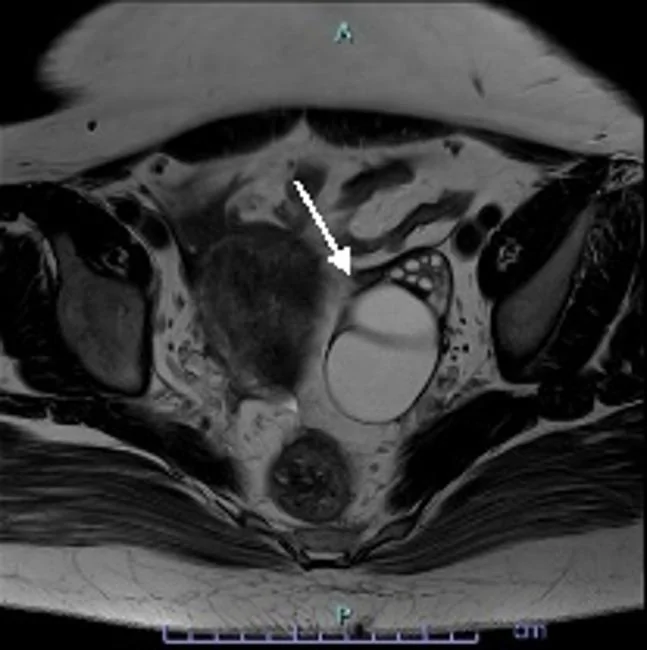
Axial T2-weighted MRI reveals a 6.5 cm multilocular tumor in the left ovary (arrow). No solid components or mural thickening are present
